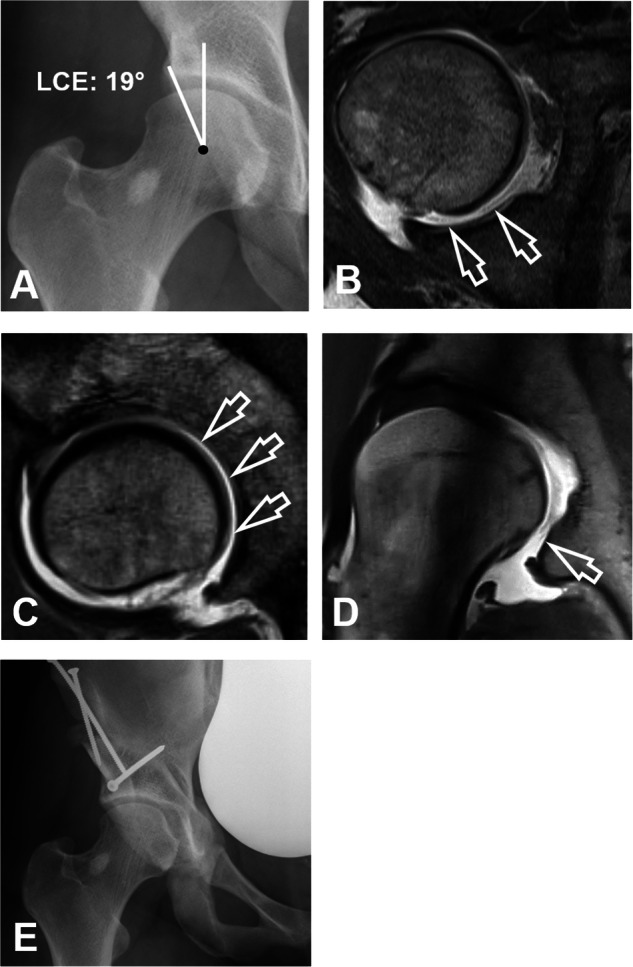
23-year-old woman with hip pain. A AP pelvis radiograph showing acetabular dysplasia with a decreased lateral center-edge (LCE) angle of 19°. The patient was referred to direct MR arthrography of the hip. B Axial-, (C) sagittal- and (D) radial proton density-weighted turbo spin echo images showing contrast interposition (arrows) between the posterior femur and the acetabulum consistent with femoral head decentration. E Postoperative AP pelvis radiograph after periacetabular osteotomy for correction of deficient acetabular coverage and hip instability
